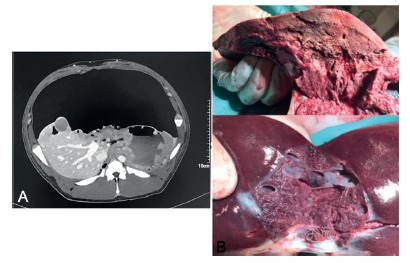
A) CT after laparoscopic hepatectomy; B) right hepatic lobe, right portal vein branch and gallbladder.
